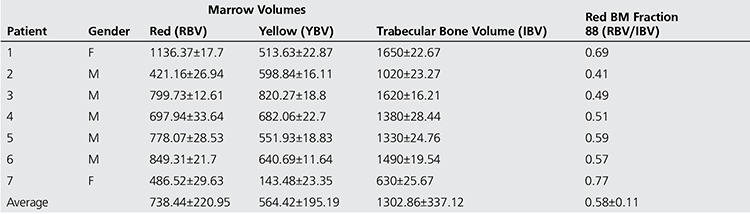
Estimated Whole Body BM volumes using 18F-FLT PET/CT imaging
